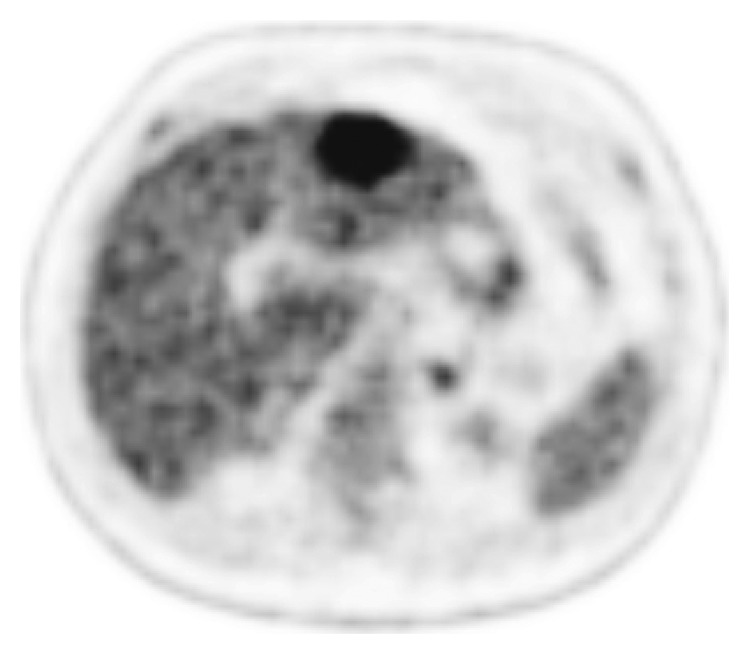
FDG PET-CT showing the hypermetabolic segment III liver lesion (SUV max: 7.3).
